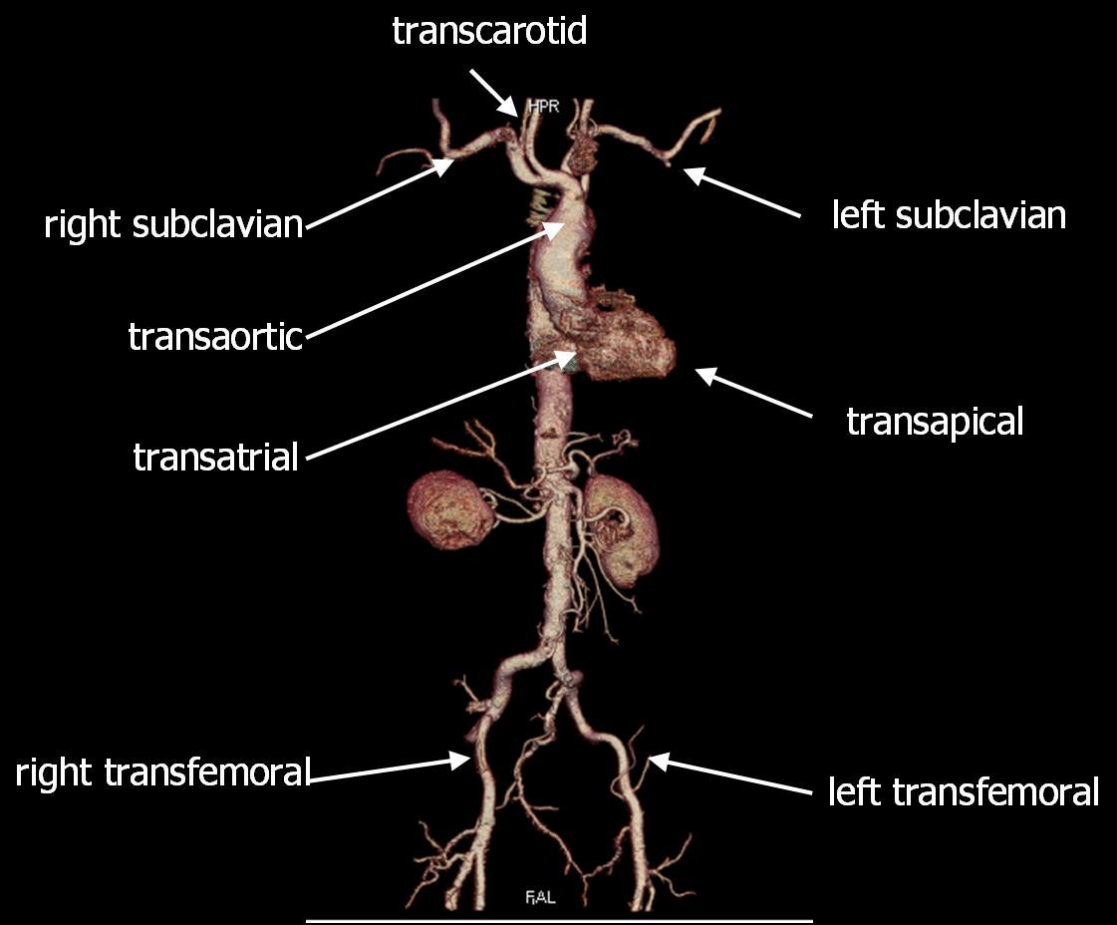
CT scan of the thoracic and abdominal aorta, demonstrating the various access options for transcatheter valve procedures.
